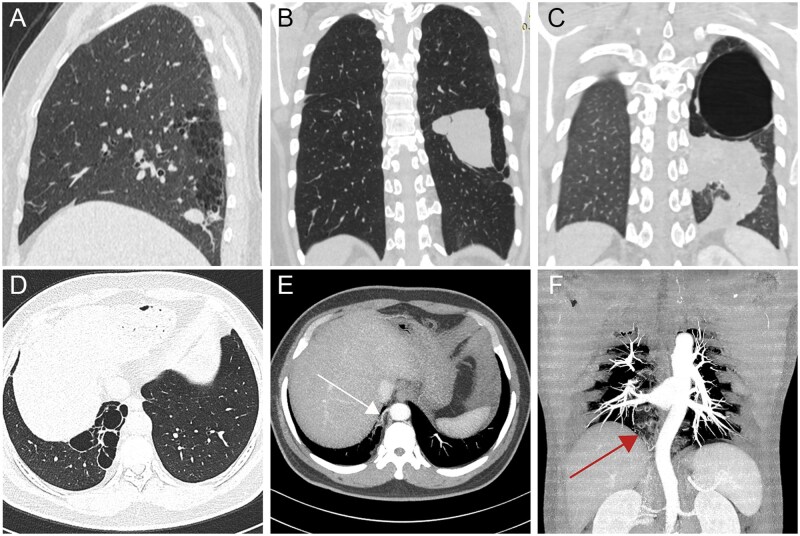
Congenital cystic lung diseases. CPAM: 63-year-old woman, multiple cysts with irregular soft-tissue mass in the right lower lobe (A); 25-year-old woman, oval soft-tissue mass in the left lower lobe (B); and 24-year-old woman, large irregular soft-tissue mass with giant pulmonary cyst in the left lung (C). PS in a 43-year-old man (D-F). Axial chest CT image (D) demonstrates a well-demarcated, air-filled, paravertebral multicystic lesion in the posterior segment of the right lower lobe. 3D reconstruction shows systemic arterial supply from a branch of the thoracic aorta (E, white arrow) and systemic venous drainage (F, red arrow). Abbreviations: CPAM = congenital pulmonary airway malformation; PS = pulmonary sequestration.
